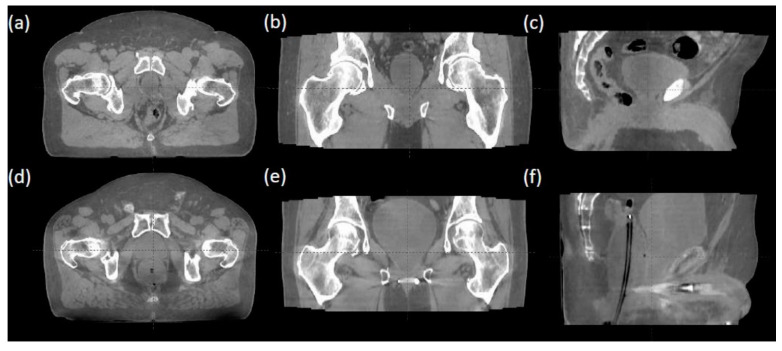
(a–c) iCBCT reconstruction of a patient without endorectal balloon. (d–f) iCBCT reconstruction of a patient with endorectal balloon.
